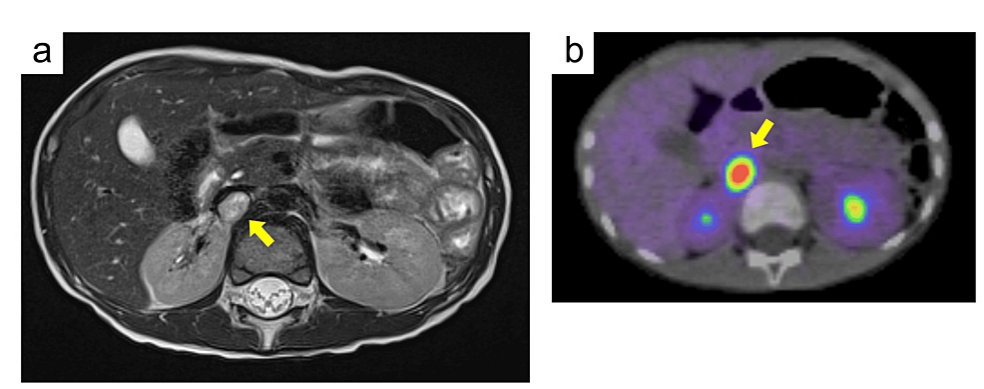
Imaging studies of the patient at initial onset Axial abdominal MRI T2-weighted images (a) and 18F-FDG-PET (b). Tumors are indicated by yellow arrows. FDG: F-18 fluorodeoxyglucose; PET: positron emission tomography
